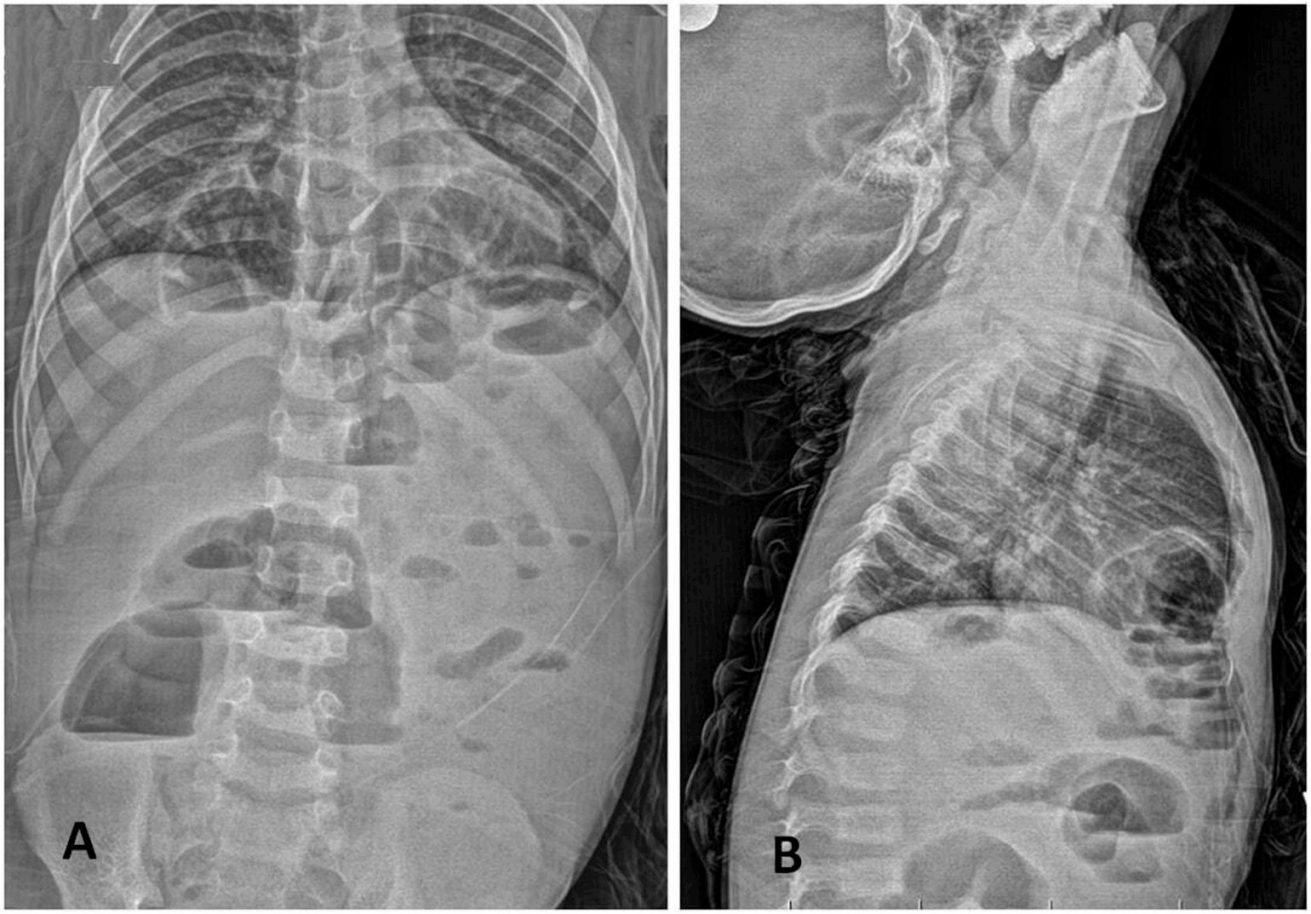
Anteroposterior (A) and lateral (B) chest x-ray showing anterior herniation of bowel loops into the chest and abdominal air fluid levels.
